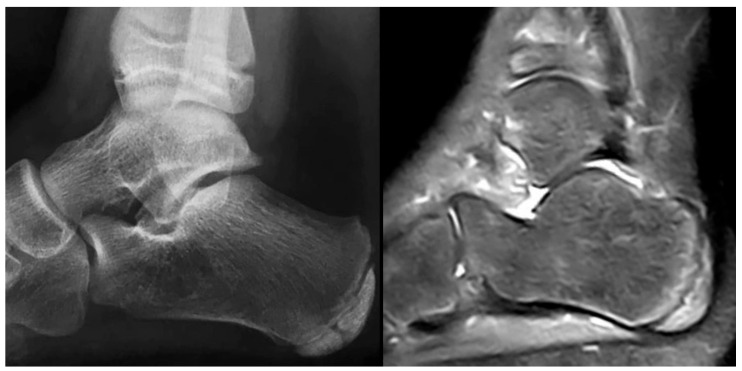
Ten-year-old male patient. Radiograph (left) revealing marked fragmentation, thinning, and bipartition of the calcaneal apophysis. MRI (right) demonstrating extensive associated bone marrow edema.
